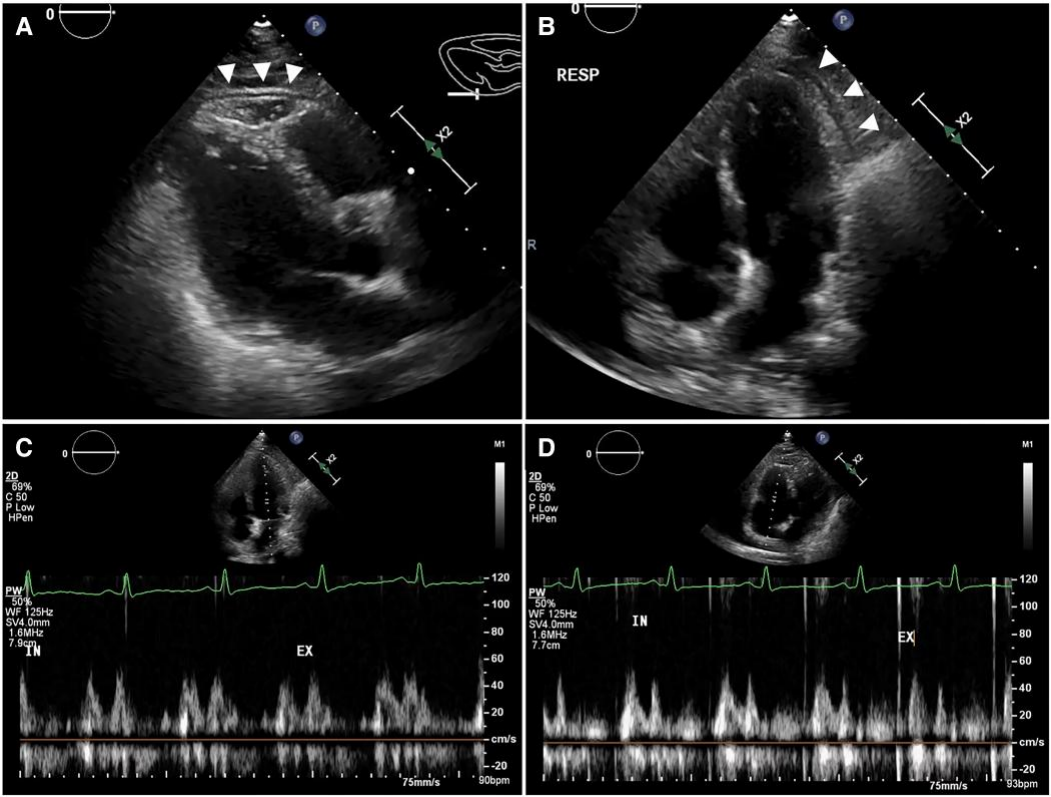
(*A*, *B*) Ultrasound echocardiography shows reduced right ventricular ejection fraction, diastolic dysfunction, and pericardial thickening (arrowheads). Respiratory variations are absent in the transmitral (*C*) and transtricupsid flow patterns (*D*).
